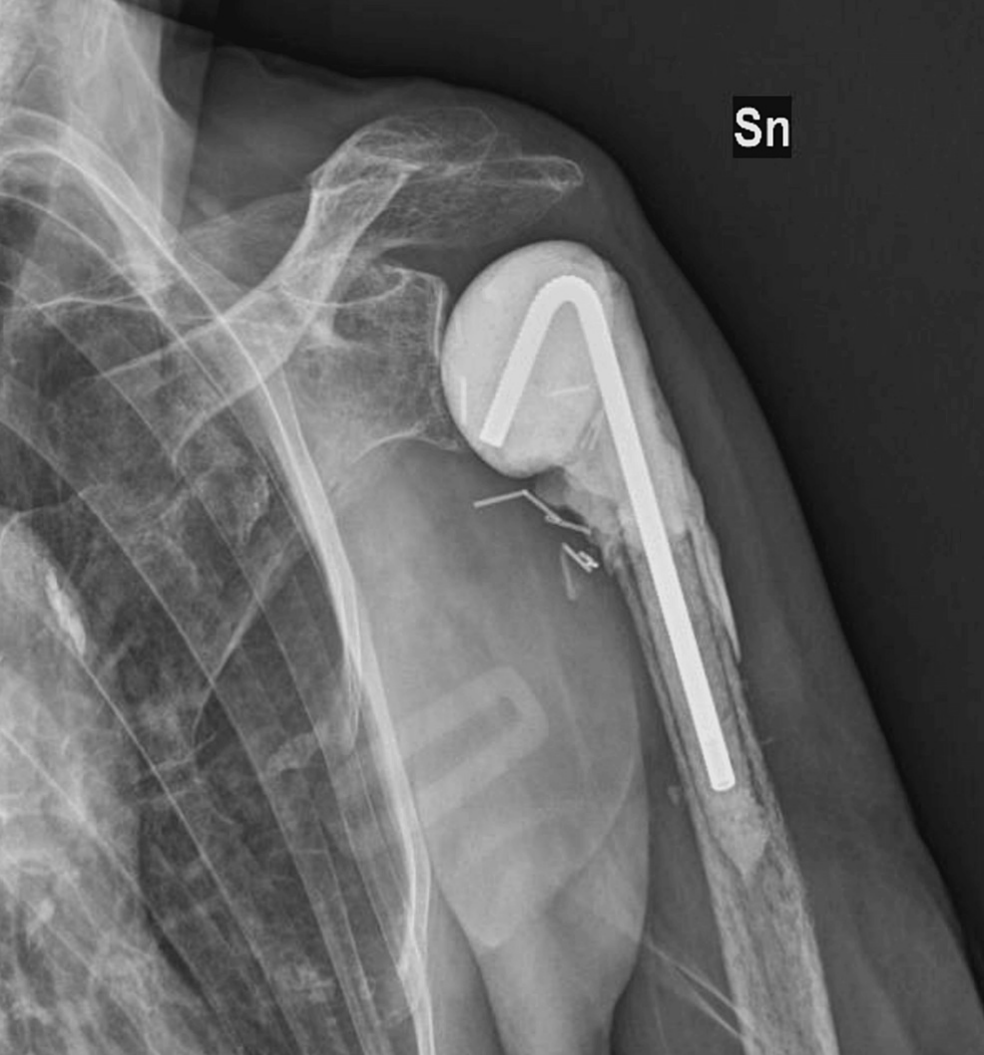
X-ray after implantation of antibiotic-loaded spacer (Case 1) Sn: left
